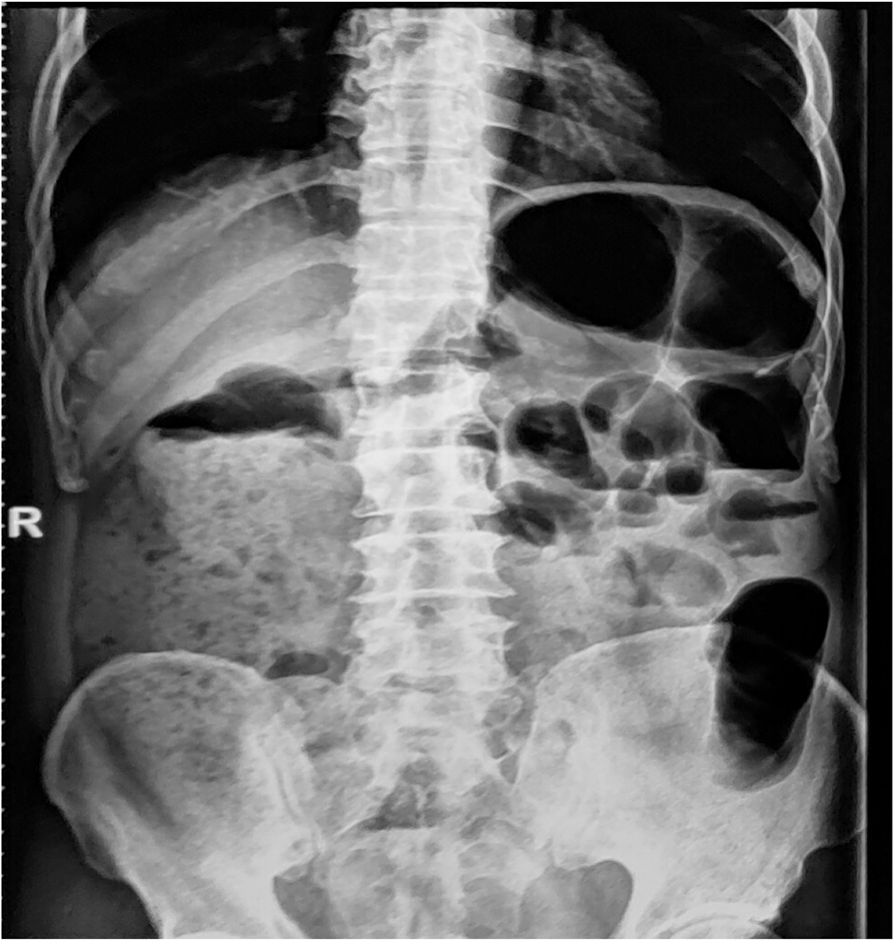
Plain X-rays and abdominal computed tomography scan demonstrated air-fluid levels and an incomplete obstruction of the colon
